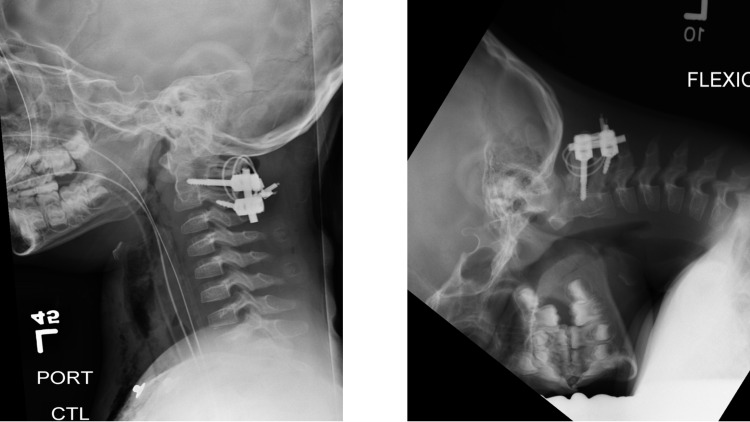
Postoperative flexion/extension X-ray two months postoperatively Imaging revealed appropriate hardware placement without any instability.
